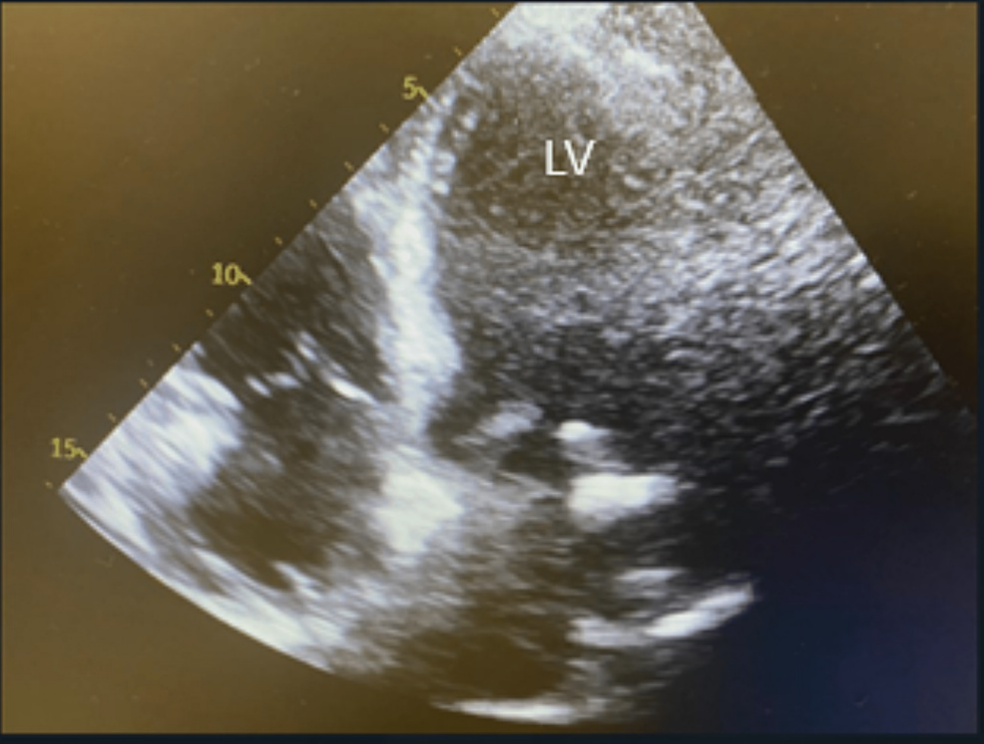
Two-dimensional echocardiography (four-chamber long-axis view) LV, left ventricle
